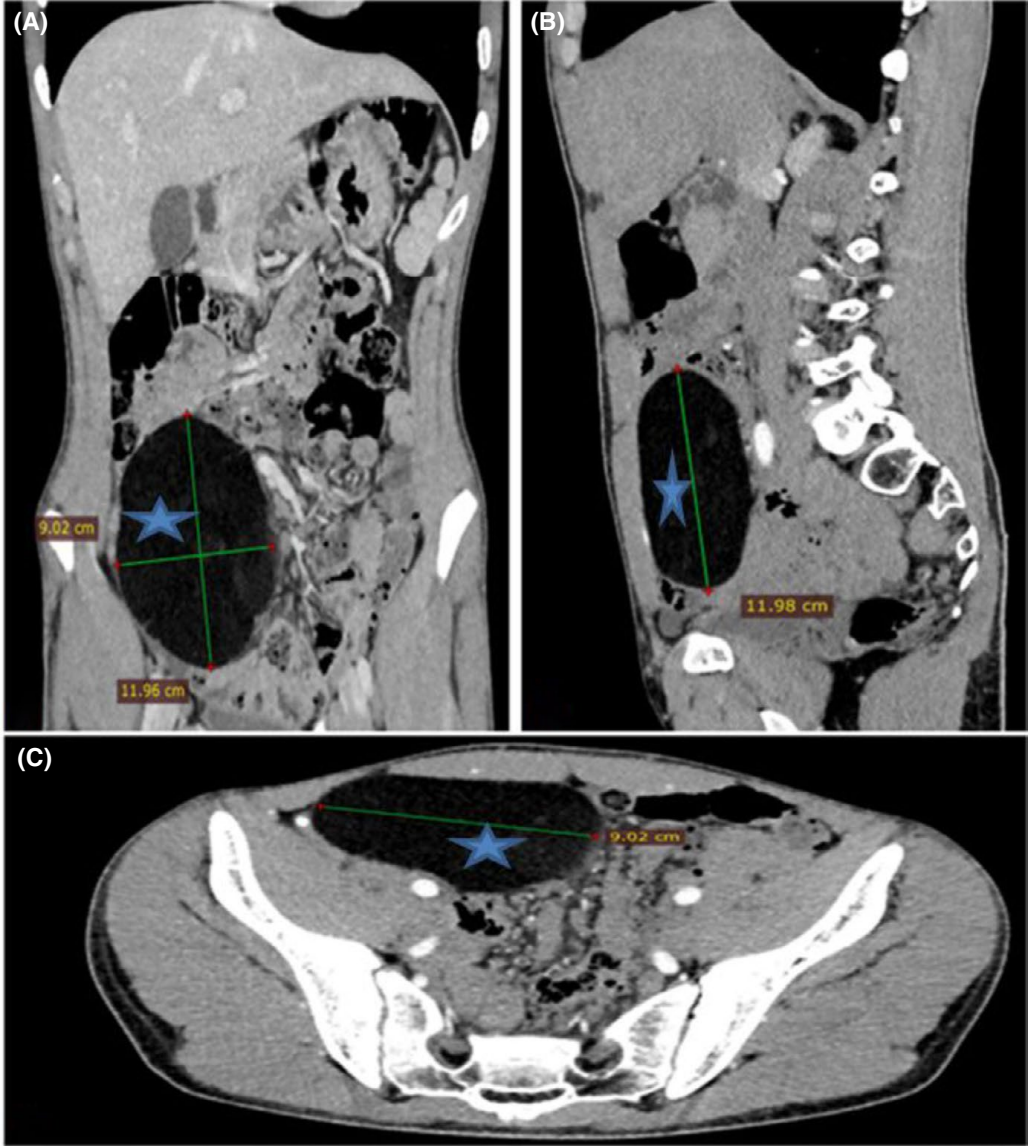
Abdominal CT scan showing mesenteric lipoma localized in the right iliac fossa measuring 12 × 9 cm (blue star)
